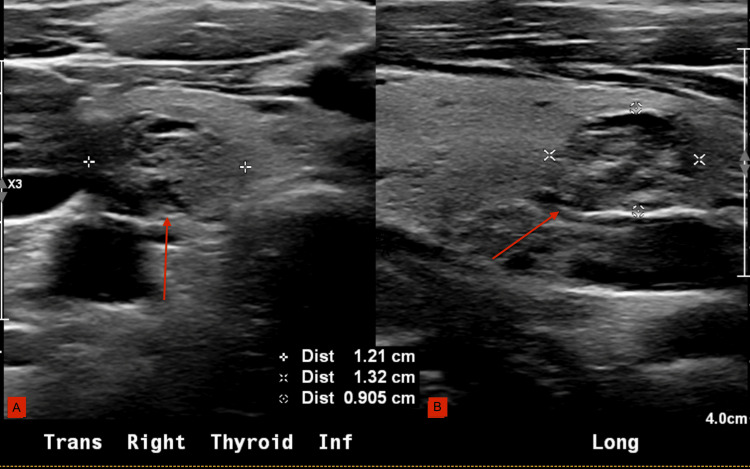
Thyroid ultrasound (13 mm right thyroid nodule) (A) Trans Mid Right Thyroid. (B) Long Mid Right Thyroid.
